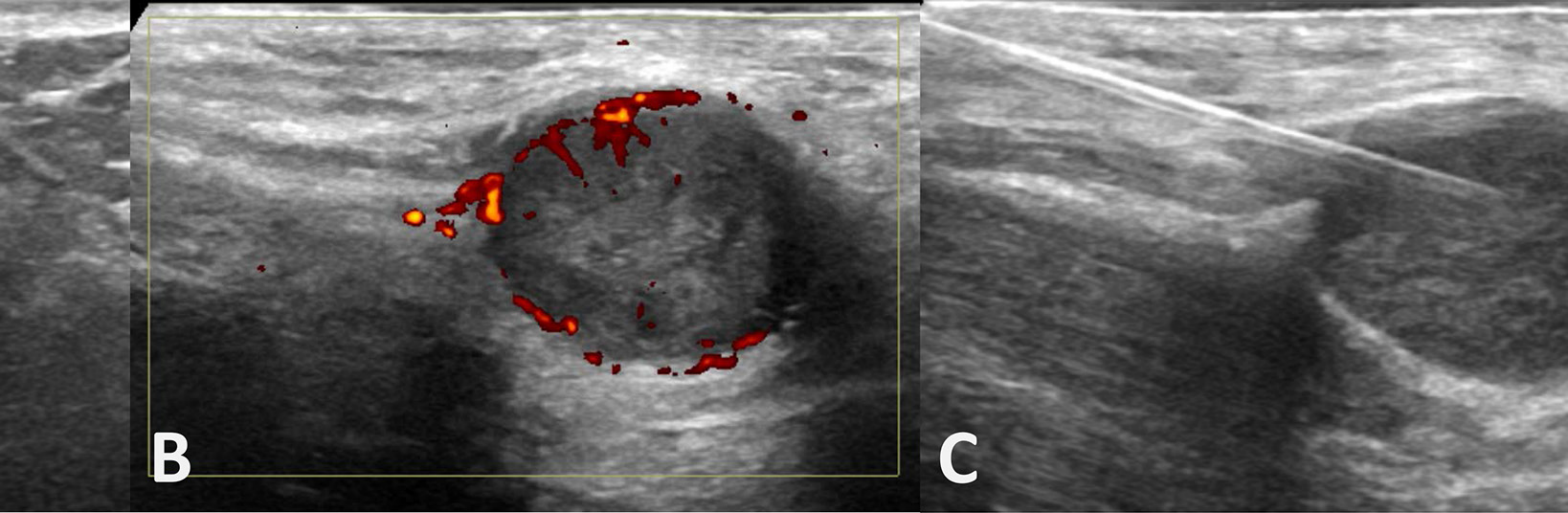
(A) Grayscale ultrasound shows a hypoechoic lesion. (B) There was posterior acoustic enhancement and peripheral power colour doppler flow. (C) Fine needle aspiration cytology of the lesion was performed under ultrasound guidance.
